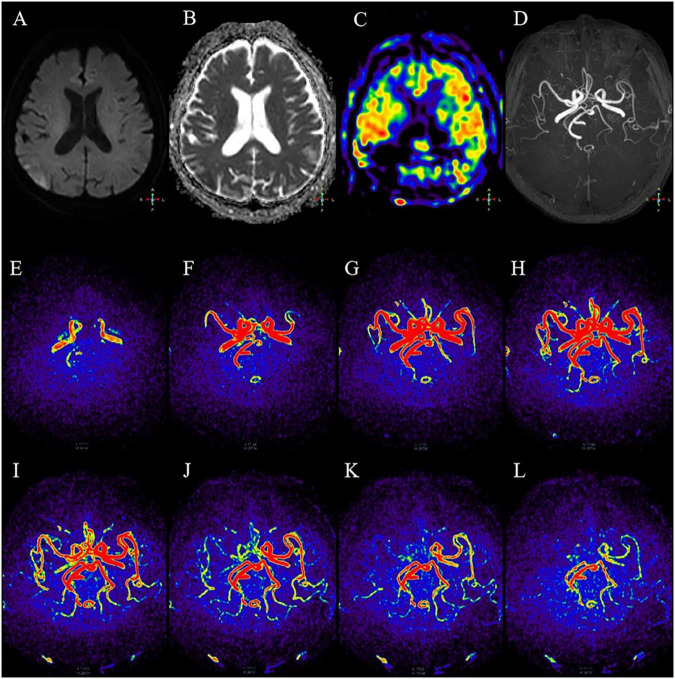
A 47-year-old male with dizziness, right limb weakness, and sensory impairment. (A,B) Local diffusion limitation in the right parietal lobe of DWI. (C) 3D-ASL showed large areas of underperfusion around the lesion. (D) No significant stenosis of the TOF-MRA cerebral artery. (E–L) According to the dynamic changes of blood flow in different periods of 4D-TRANCE, the right middle cerebral artery flowed faster than the left side. With an ischemic penumbra, bilateral blood flow velocity was discordant.
